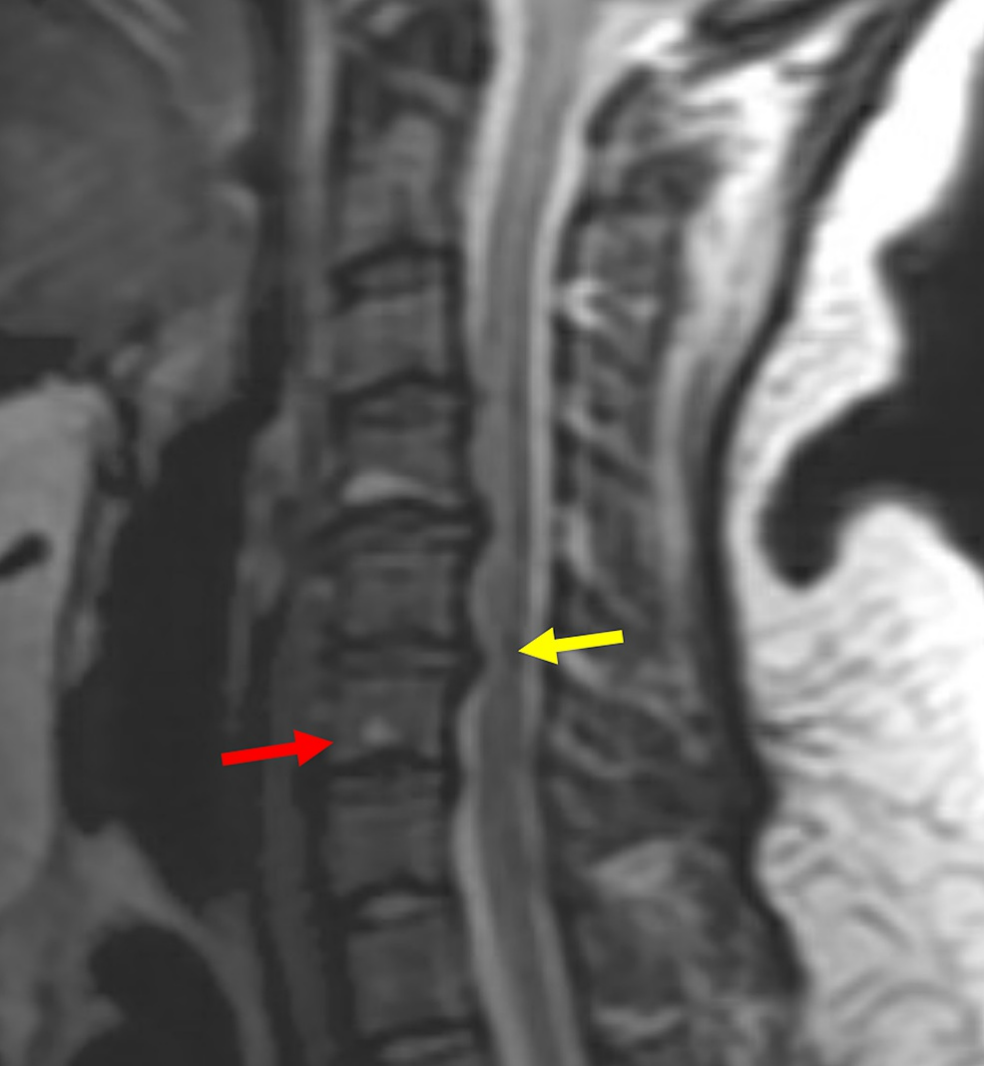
MRI showing cervical spondylosis at C3-C7 abutting the spinal cord (yellow arrow) and enhancing bony lesion at the C6 vertebrae (red arrow).
